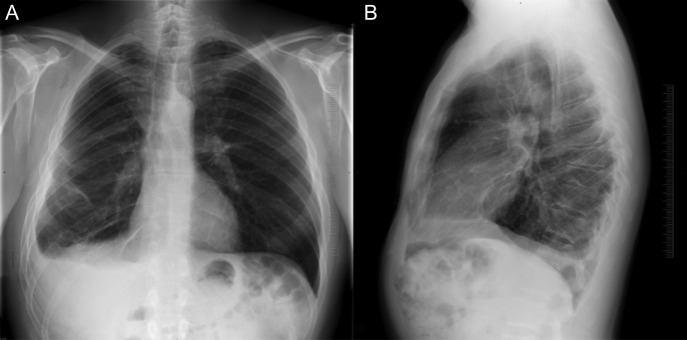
Chest X-ray in antero-posterior (A) and lateral view (B) showing consistent reduction of the right lung with basal pleural effusion.
